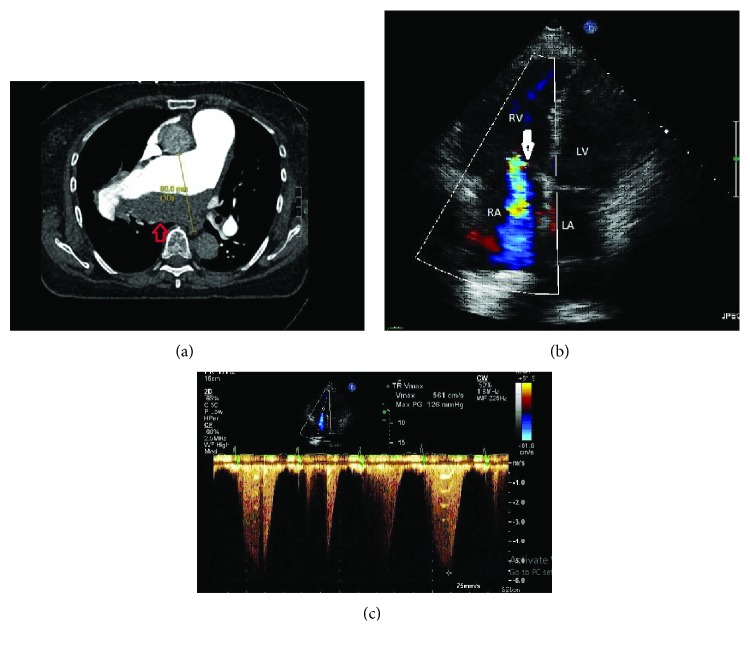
(a) CTA chest showing right pulmonary artery aneurysm measuring 80 mm in the greatest dimension with laminated thrombus in the aneurysmal sac (red arrow). (b) Transthoracic echocardiogram apical four-chamber view showing dilated RV, severe TR (white arrow), and systolic septal flattening consistent with RV pressure overload. (c) Extremely elevated estimated PA systolic pressure measurements to 134 mmHg indicating severe PH.
